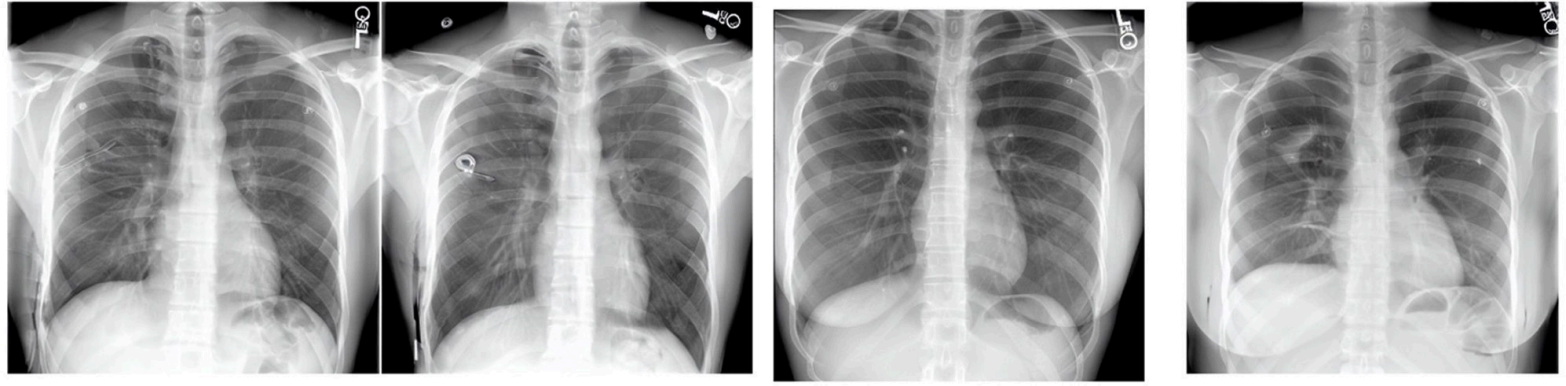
The output of Roentgen with the left two images representing “pneumothorax with chest tubes” and the right two images “pneumothorax without chest tubes”. Visual inspection shows fragmented chest drains and no obvious pneumothorax on the images without chest tubes.
